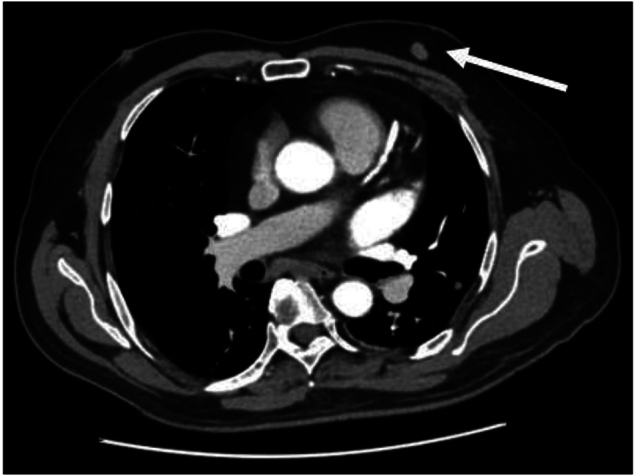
Computed tomography scan showing a 12mm left anterior chest wall lesion
